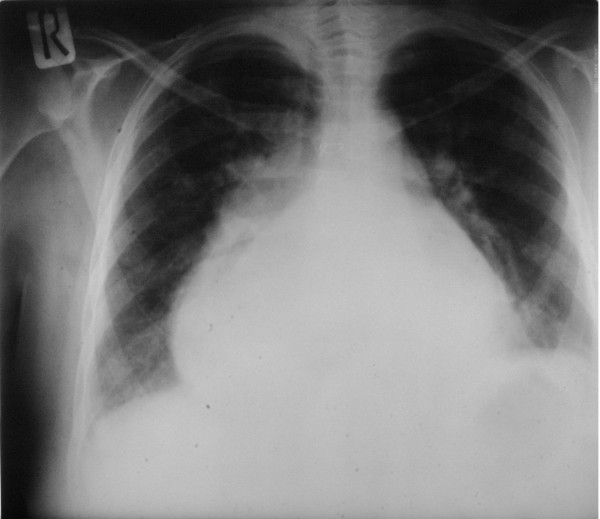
Postoperative chest x-ray showing reduction of cardiothoracic ratio.
